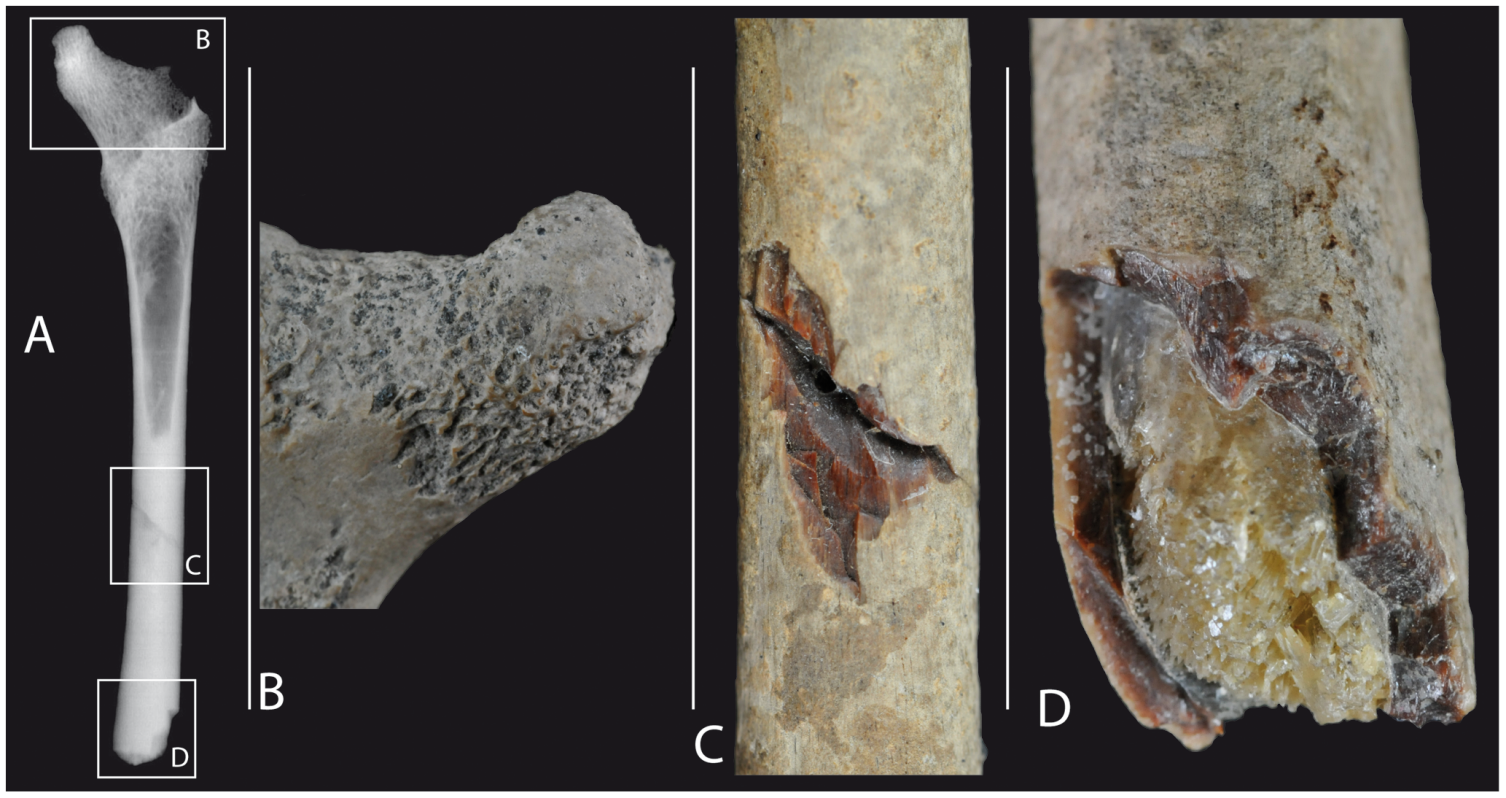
Taphonomic details of Trinil 5703. A: radiograph in anterior view showing an opaque filling in the distal portion of the preserved medullar cavity; B: detail of the neck in posterior view, showing fluvial abrasion in weakly acid conditions; C: detail of the refitted break of the shaft that we attribute to breakage during excavation; D: detail of the distal break of the shaft, which is likely also excavation damage, and the calcitic filling inside the medullar cavity, as also seen on the radiograph.
